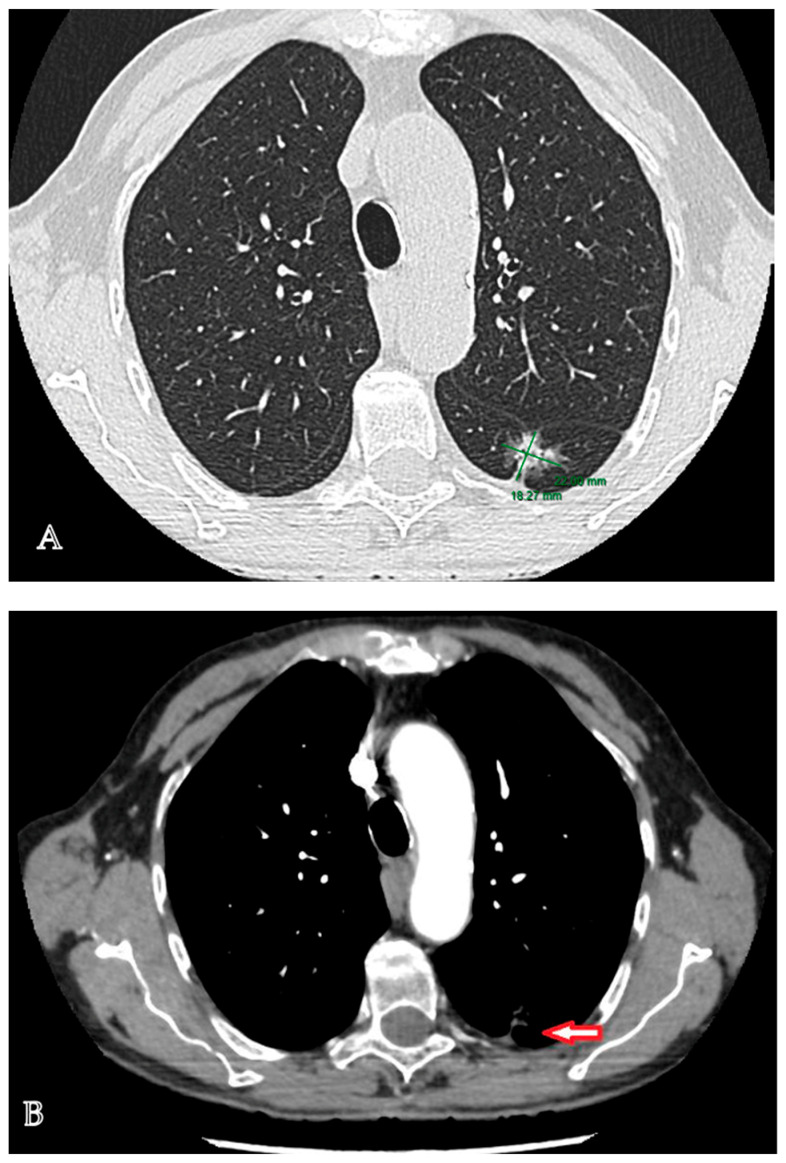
Lung adenocarcinoma located at the level of the left Fowler segment. (A). Contrast-enhanced chest computed tomography: a partially solid spiculiform tumor with maximum dimensions of 22/18/20 mm, located in the left Fowler segment. (B). Contrast-enhanced chest computed tomography, arterial phase: a partially solid spiculiform tumor that sends extensions to the parietal pleura and oblique fissure, exerting a retractive effect on both structures.
